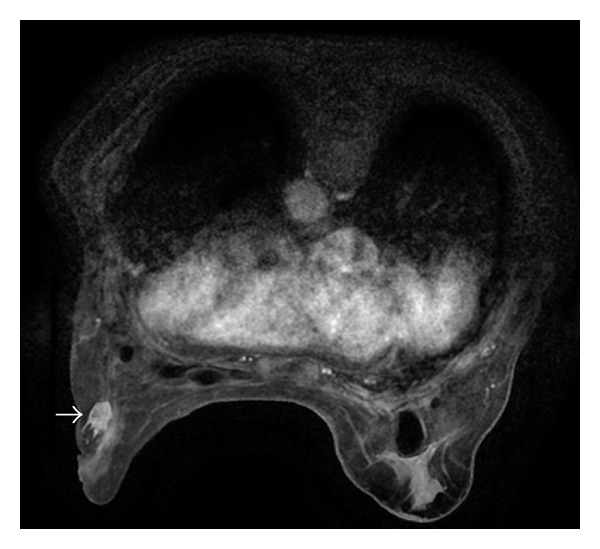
Axial fat-suppressed postcontrast breast MRI shows a 3 cm enhanced irregular mass with focal irregular (white arrow) in the left breast under compression before MRI-guided biopsy. It was diagnosed as ILC by the biopsy.
